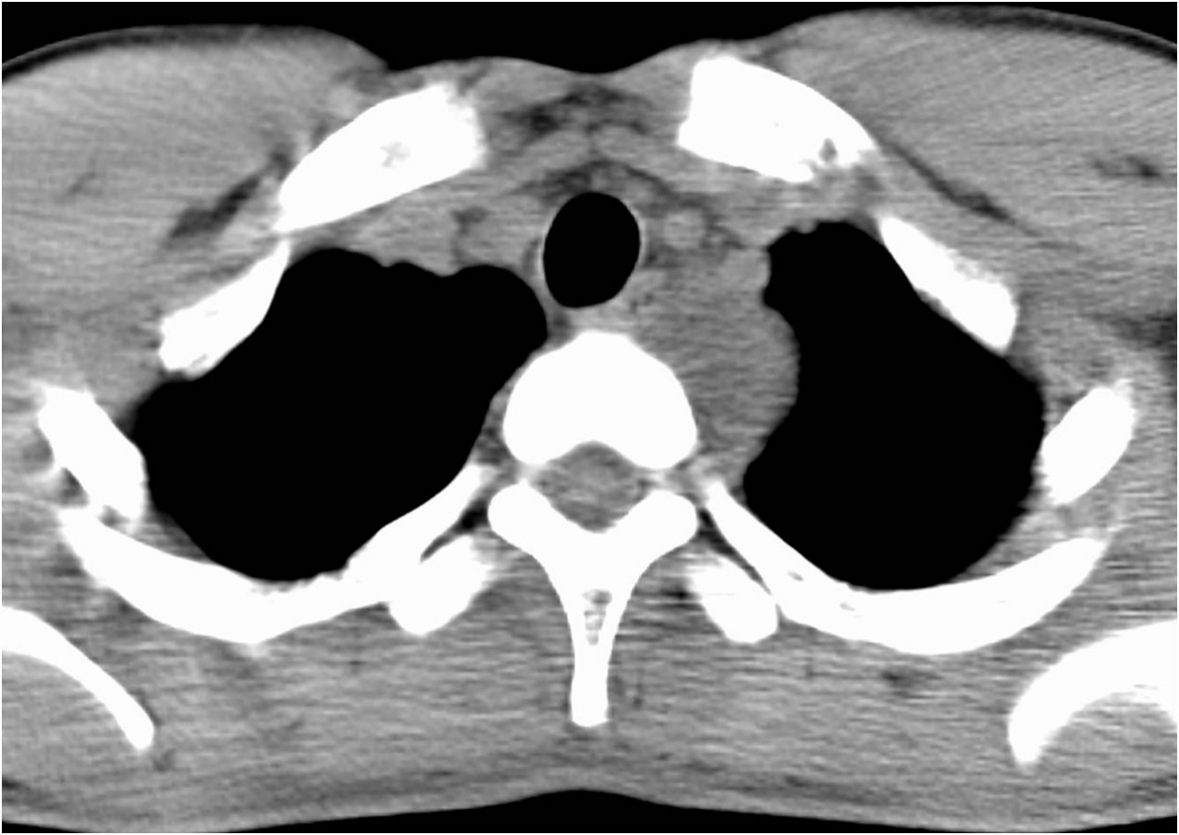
Chest computed tomography showing a 36 × 21 × 30 mm superior mediastinal mass located between the left common carotid and subclavian arteries, trachea, and spine.
